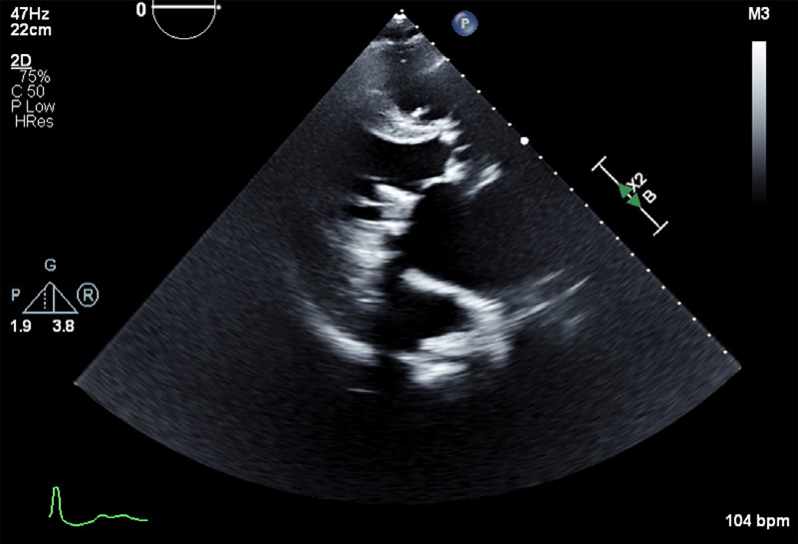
Transthoracic Echocardiogram Parasternal long-axis view showing resolution of pericardial effusion after pericardiocentesis and pigtail catheter placement.
